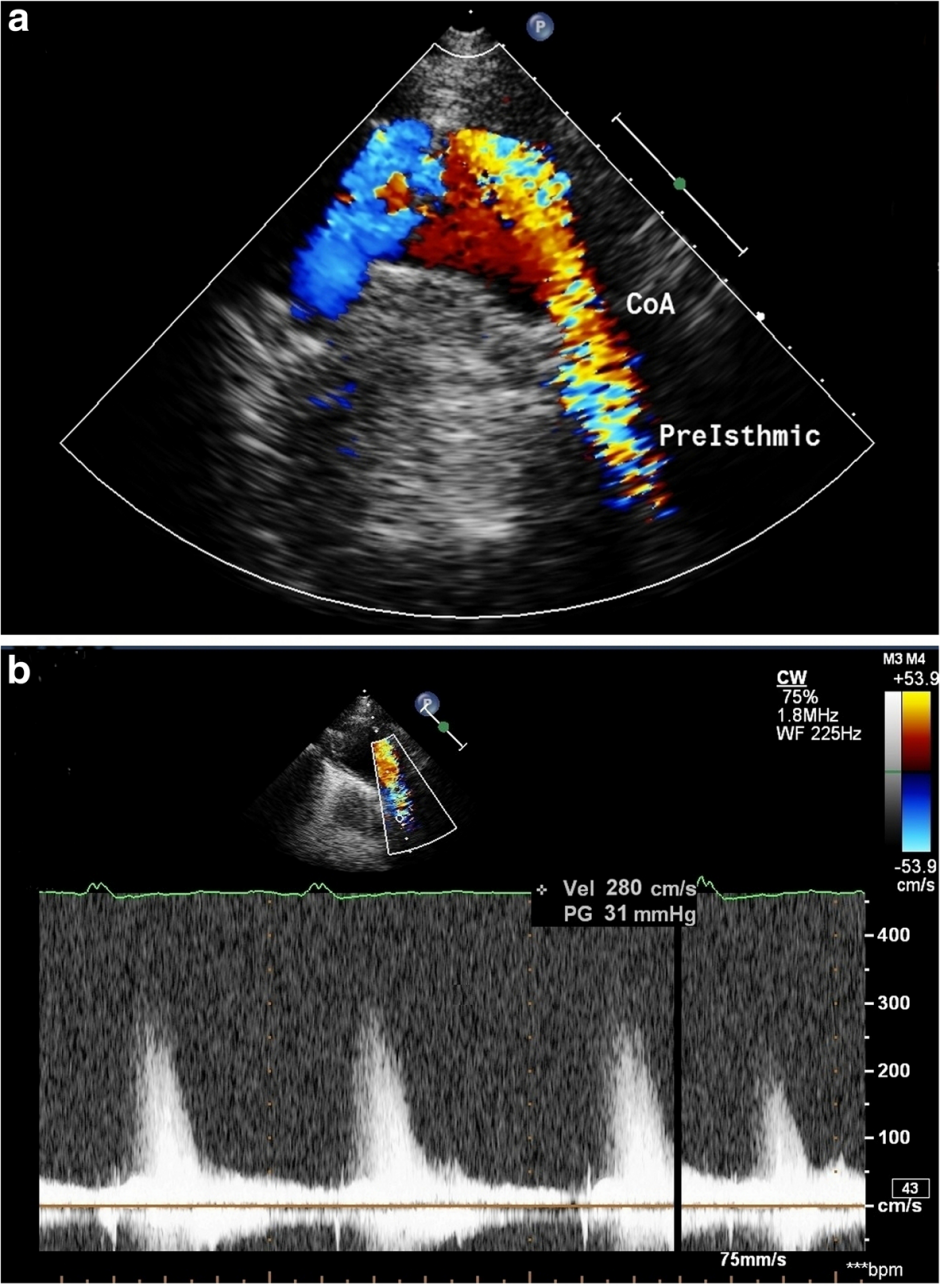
Transthoracic echocardiography through dorsal approach. Color Doppler flow acceleration across the CoA (a); significant trans-stenosis pressure gradient (b).
